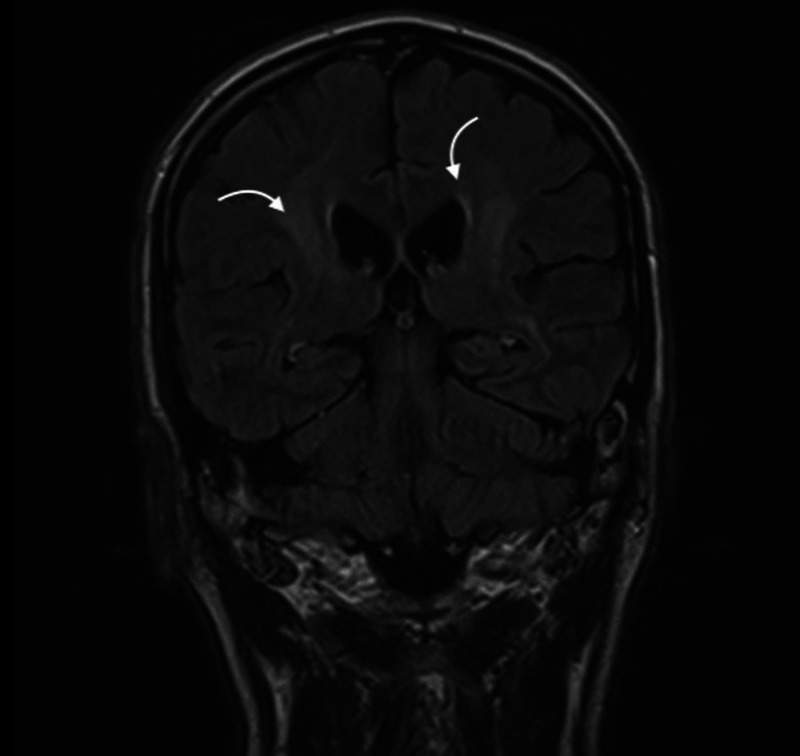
MRI demonstrating abnormal non-specific T2 hyperintense and mild FLAIR hyperintense signals. FLAIR, fluid-attenuated inversion recovery
